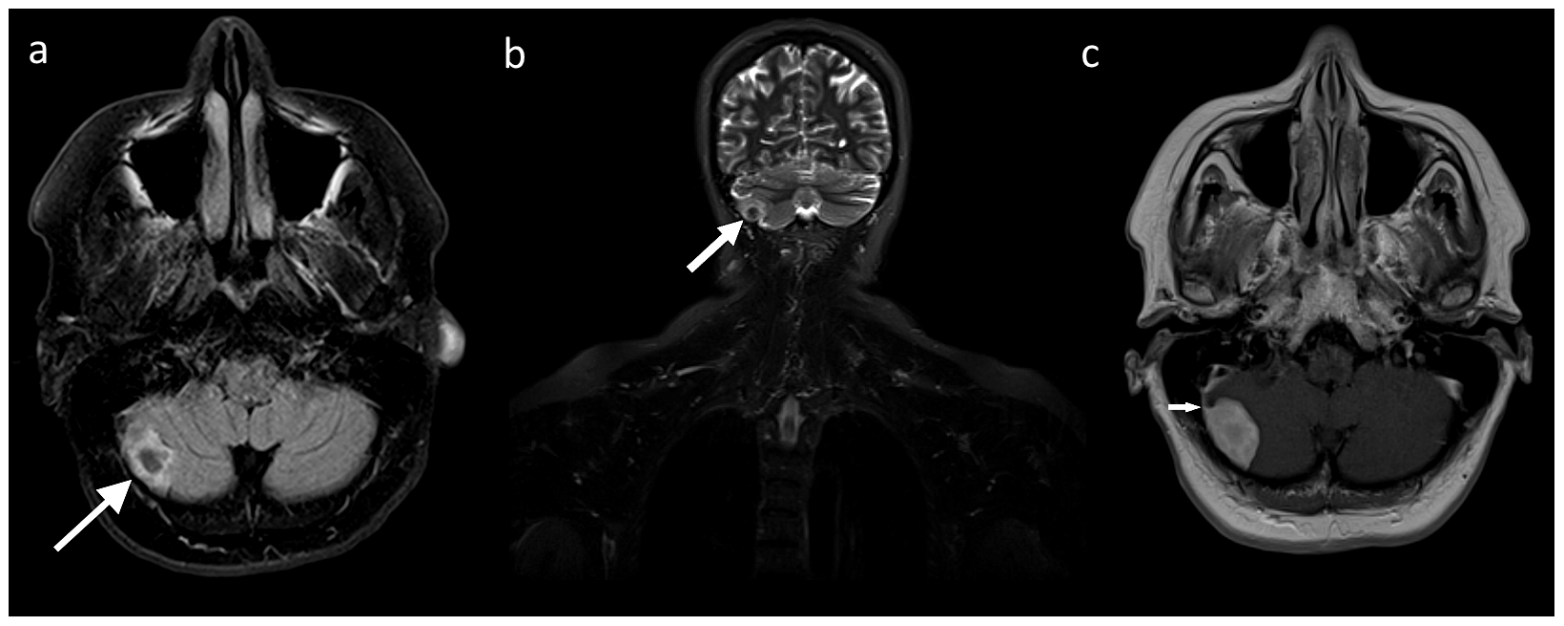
A 59-year-old female with surgically removed meningioma. A lesion of mixed signal intensity (arrows) is seen in the posterior fossa on a T2-FLAIR image of the head (A) and a coronal whole-body T2-STIR image (B). A supplementary, preoperative contrast-enhanced MR study (C) demonstrated enhancing lesion with a tail sign (arrow).
